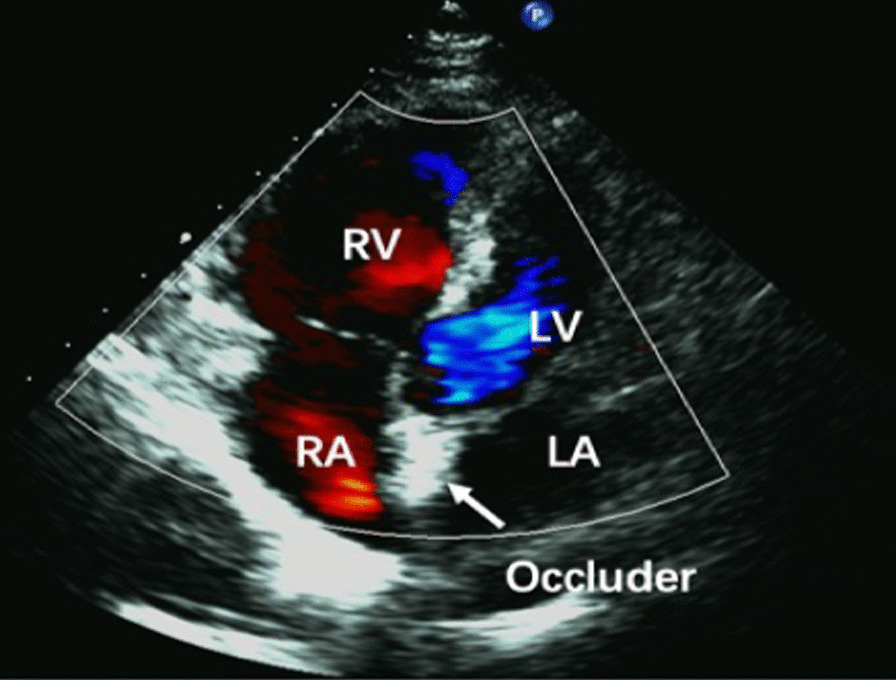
Transthoracic echocardiography shows blood flow after PFO occlusion. No residual shunt is noted between the LA and RA. *LV* left ventricular, *LA* left atrial, *RV* right ventricular, *RA* right atrial, *PFO* patent foramen ovale
